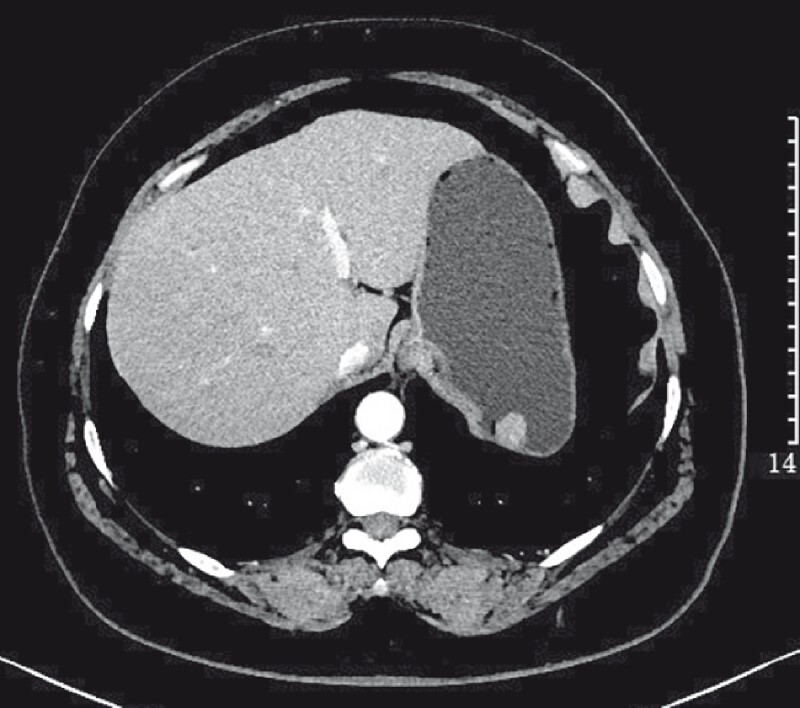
Computed tomography image showing a stromal tumor (about 2 × 1 cm) at the gastric fundus.
